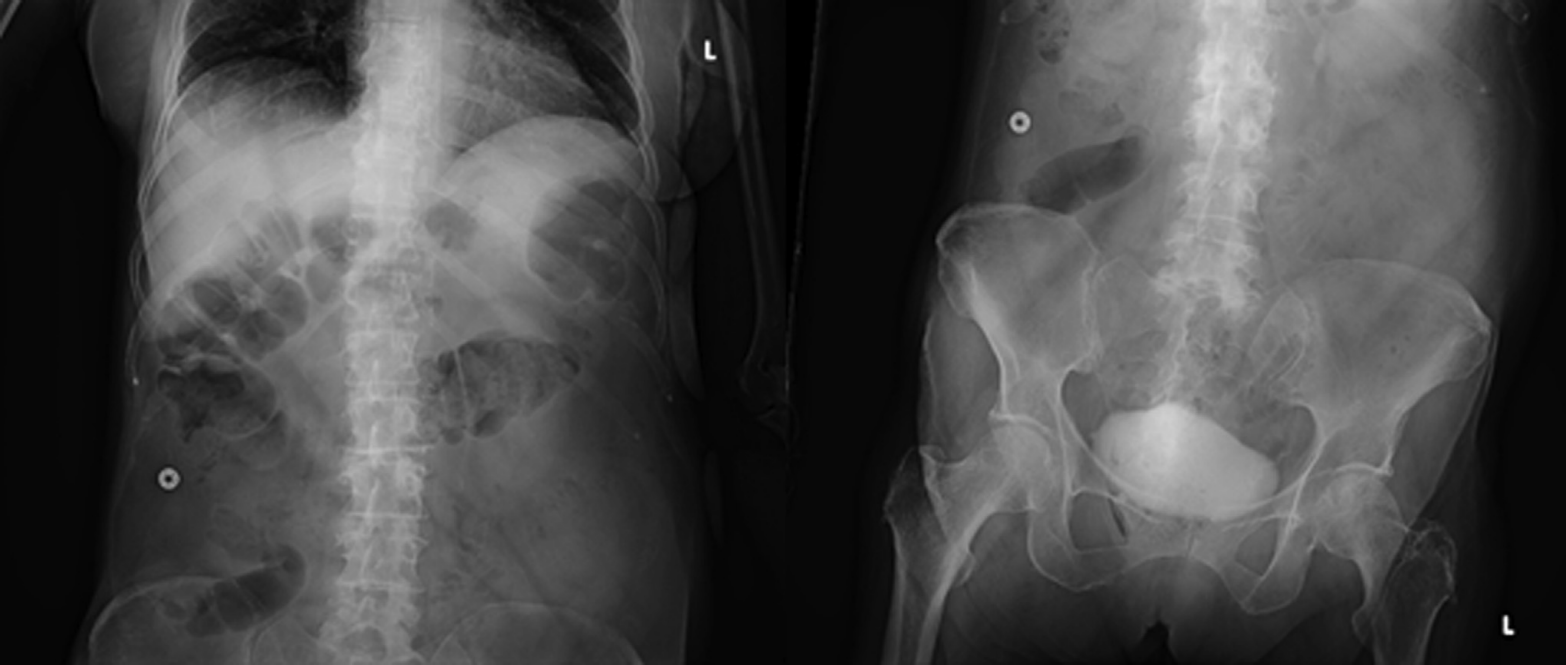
A follow-up abdominal radiograph confirming swallowed optical sensor capsule excretion.
